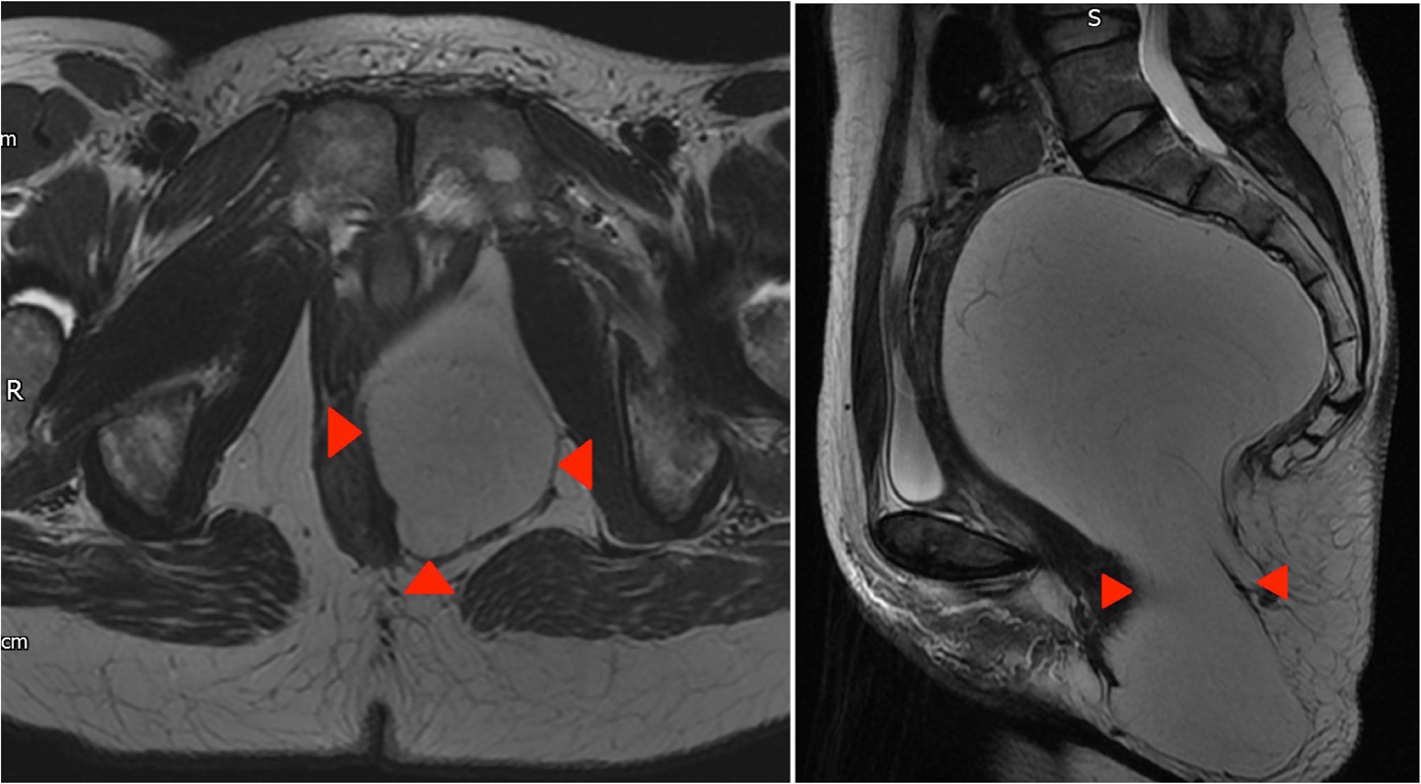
Left panel: Axial T2-weighted MR of the pelvis: arrowheads outline a fatty mass in the left ischiorectal fossa. Right panel: Sagittal T1-weighted MR image of the pelvis: large fatty pelvic lesion seen with extension through the left ischiorectal fossa into the perineal tissues. Arrowheads outline the level of the ischiorectal compartment
